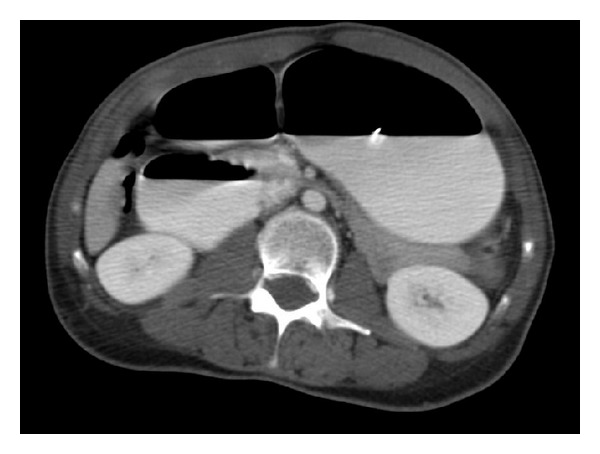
CT-imaging demonstrating SMA syndrome with resulting compression of duodenum due to aorta and superior mesenteric artery.
